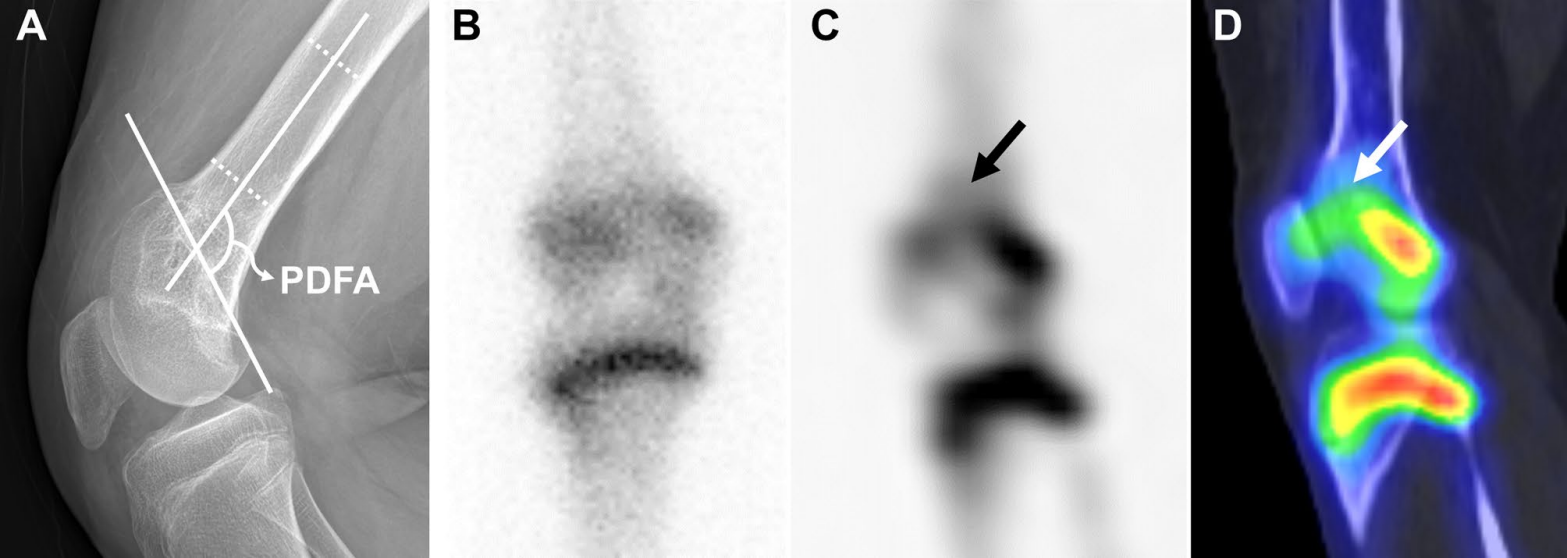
Advantage of bone SPECT/CT for assessing physeal status in which sagittal plane deformity is apparent. A 10.7-year-old girl presented with genu recurvatum after right distal femoral physeal fracture. Extension deformity of the distal femur is identified with a an anatomic posterior distal femoral angle of 125°, although the precise physeal location is obscure on conventional radiography (A). On anterior view planar scintigraphy, an overall decrease in distal femoral physeal activity is apparent. (B). However, hypoactivity is limited to the anterior distal femoral physis on the sagittal SPECT image, which has caused extension deformity (arrows) (C). It is more clearly visualized on the sagittal fused SPECT/CT (D). PDFA = posterior distal femoral angle
